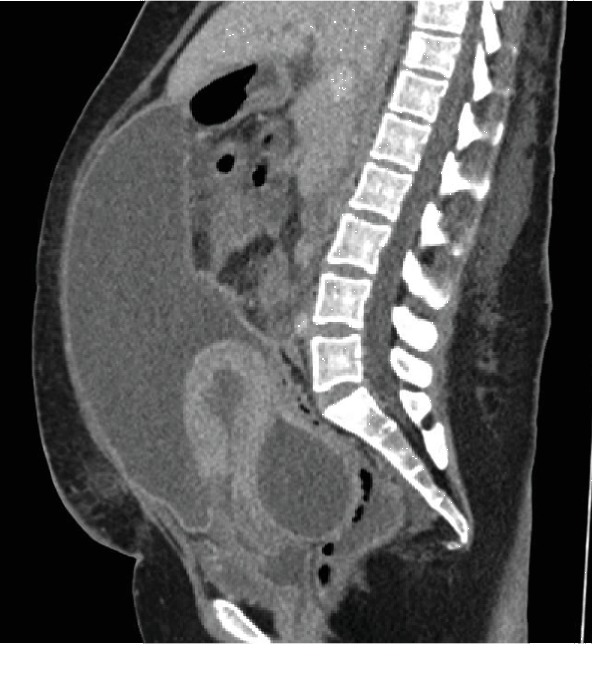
Initial CT findings of a dehisced CS scar and increase in size of the loculated ascites.
